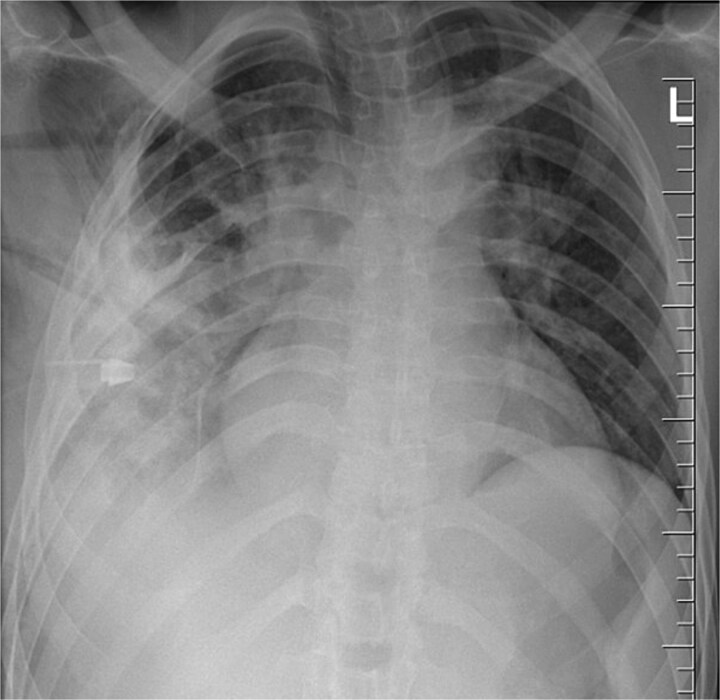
Chest radiograph post-operatively, demonstrating a chest tube in situ, residual lung opacification, and expected post-surgical changes following removal of a retained thoracic foreign body (knife).
